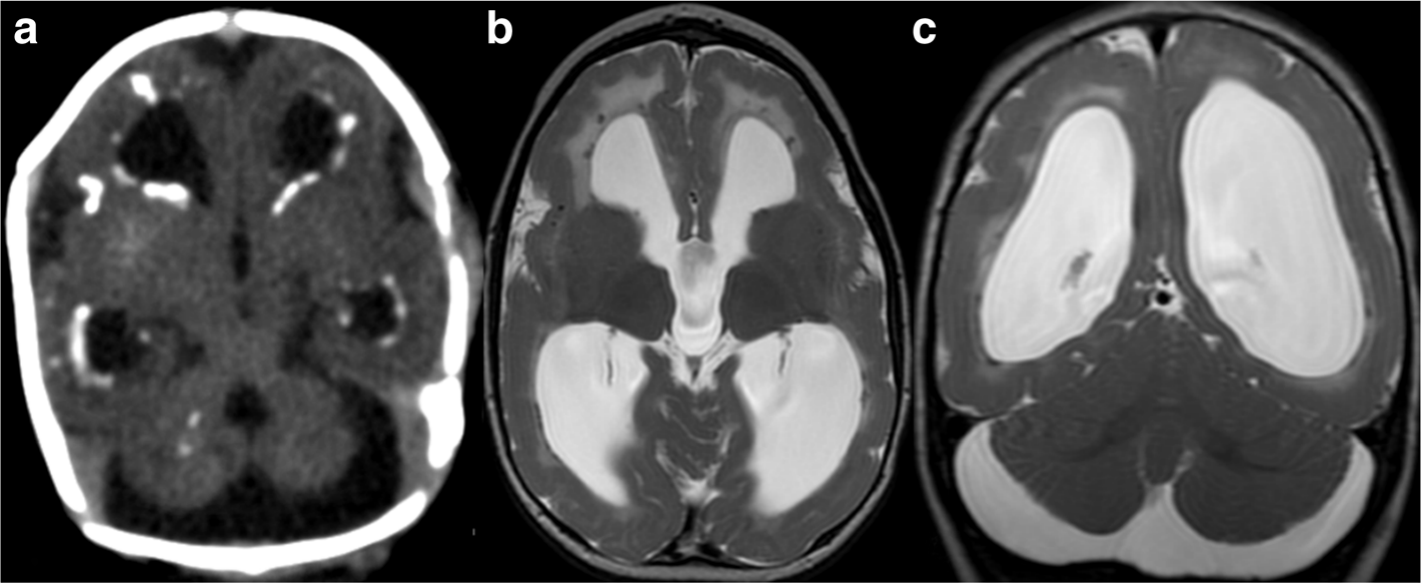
a, Axial computed tomography (CT) image of a 2-day-old male neonate with confirmed congenital cytomegalovirus infection shows ventriculomegaly, cerebellar hypoplasia, and periventricular hyperdense calcifications. b, Axial and c, Coronal T2-weighted MR images of the same child at the age of 14 months reveal cerebellar hypoplasia, ventriculomegaly, hyperintense signal of the periventricular white matter, hypointense periventricular calcifications, and diffuse polymicrogyria and pachygyria (reprinted with permission form Poretti A et al., Eur J Paediatr Neurol, 2009;13:397–407)
